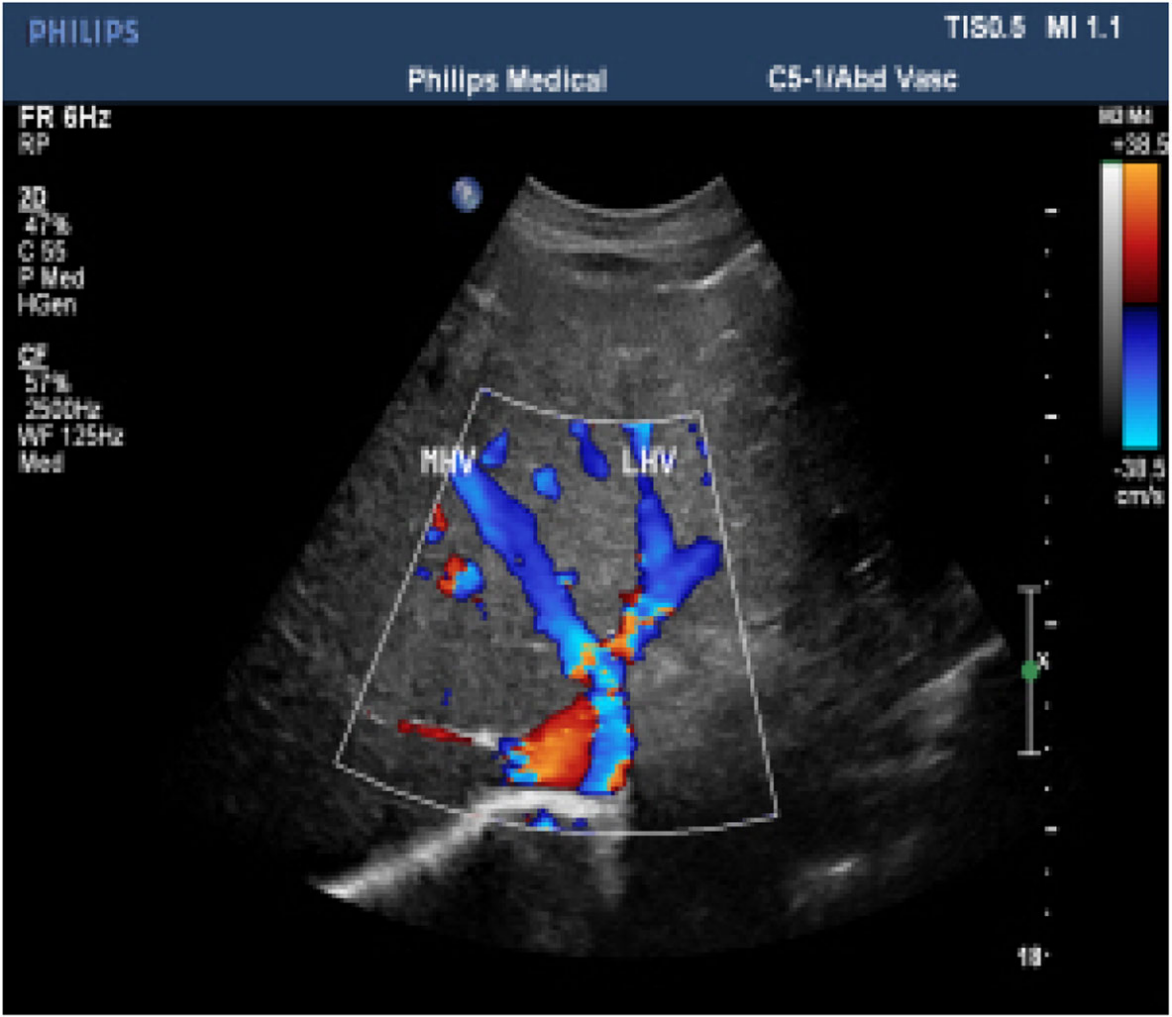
B-ultrasound of the abdomen: Ultrasound shows the left hepatic vein (blood-flow signal is narrow and disordered) and right hepatic vein (blood-flow signal was narrow) are narrow, and the middle hepatic vein is occluded (blood-flow signal is interrupted in the proximal vena cava; the distal end is dilated)
